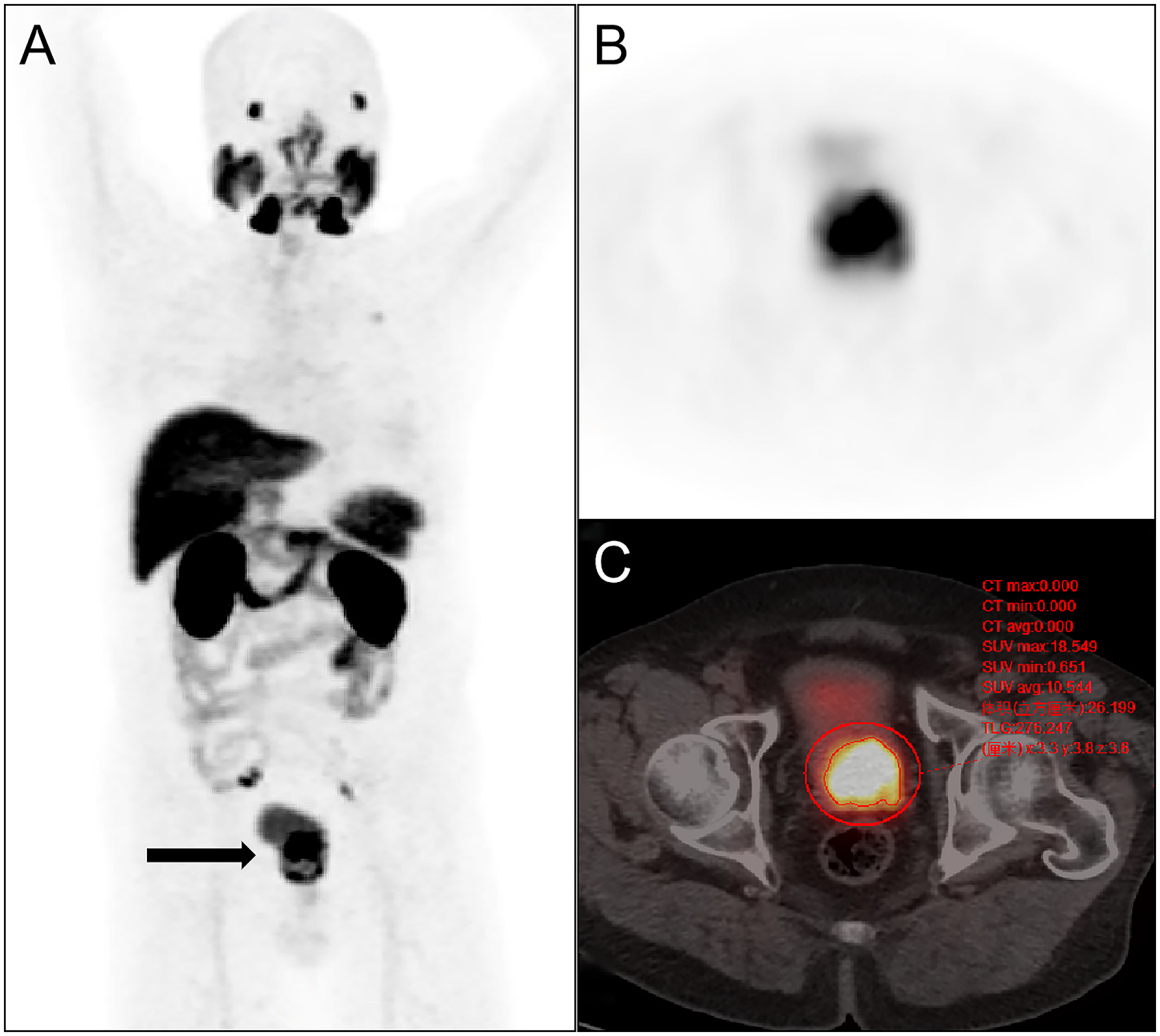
Semi-quantitative parameters of the primary prostate tumor were measured on 18F-PSMA-1007 PET/CT imaging by the 3D sketching method. (A) Primary prostate cancer was revealed on whole-body maximum intensity projection (MIP) imaging (arrow); (B) the prostate positive lesion was shown on axial PET imaging; (C) the volume of interest of the prostate lesion was obtained on axial fusion image (smaller red circle surrounding the lesion). SUVmax, TL-PSMAp, and PSMA-TVp of the lesion were obtained by the threshold method as 18.549,276.247 and 26.199 cm^3^.
